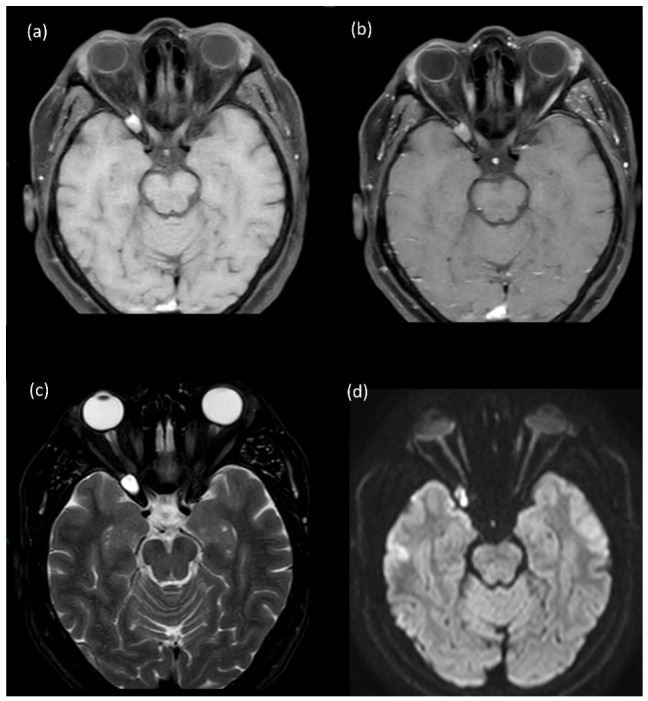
Case 3. Axial MRI demonstrating a right orbital apex lesion measuring 11.5 × 7.1 × 11.3 mm. (a) Post-contrast T1-weighted image showing notable enhancement of the lesion. (b) Fat-suppressed post-contrast T1-weighted image confirming lesion enhancement. (c) T2-weighted image delineating lesion extent. (d) T2-FLAIR image demonstrating a solid lesion with surrounding signal alteration.
